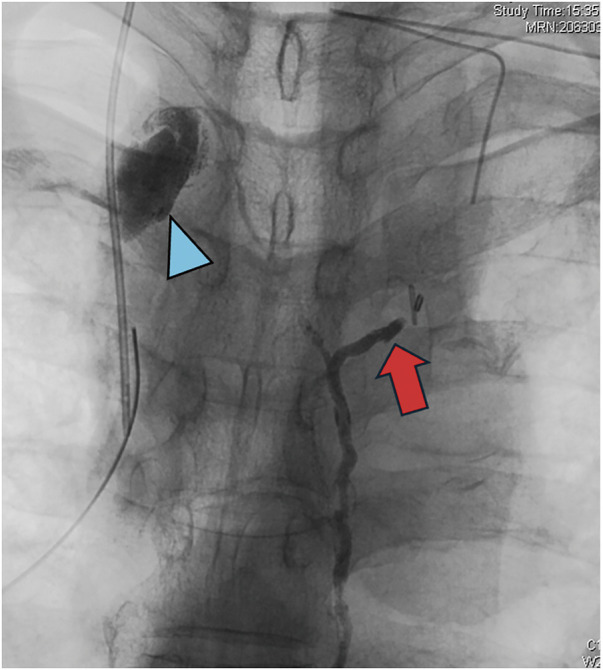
Upper mediastinal X-ray image during lymphangiography performed on POD14. The image shows the ligated left branch of the thoracic duct (arrow) from the initial surgery and contrast agent leakage from the stump of the right branch of the thoracic duct (arrowhead).
